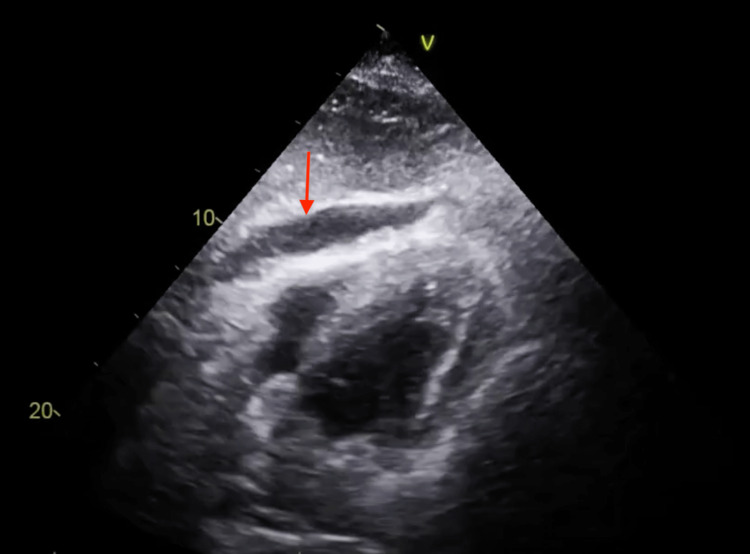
Echocardiogram subcostal view showing large pericardial effusion (arrow) with evidence of tamponade physiology
